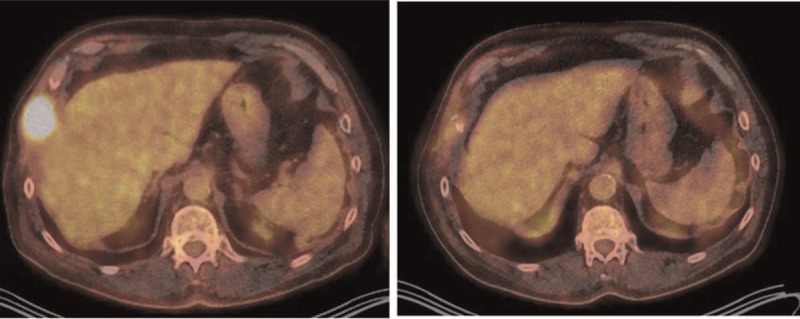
18F-PET/CT: Left image—A recurrence in the stump of the 8th rib in September 2018; Right image—After the 2nd radioiodine therapy and external beam radiotherapy, a regression of the recurrence in the stump of the 8th rib was seen in February 2019. Unfortunately, this regression was only temporary, so treatment with sorafenib was initiated in April 2019.
